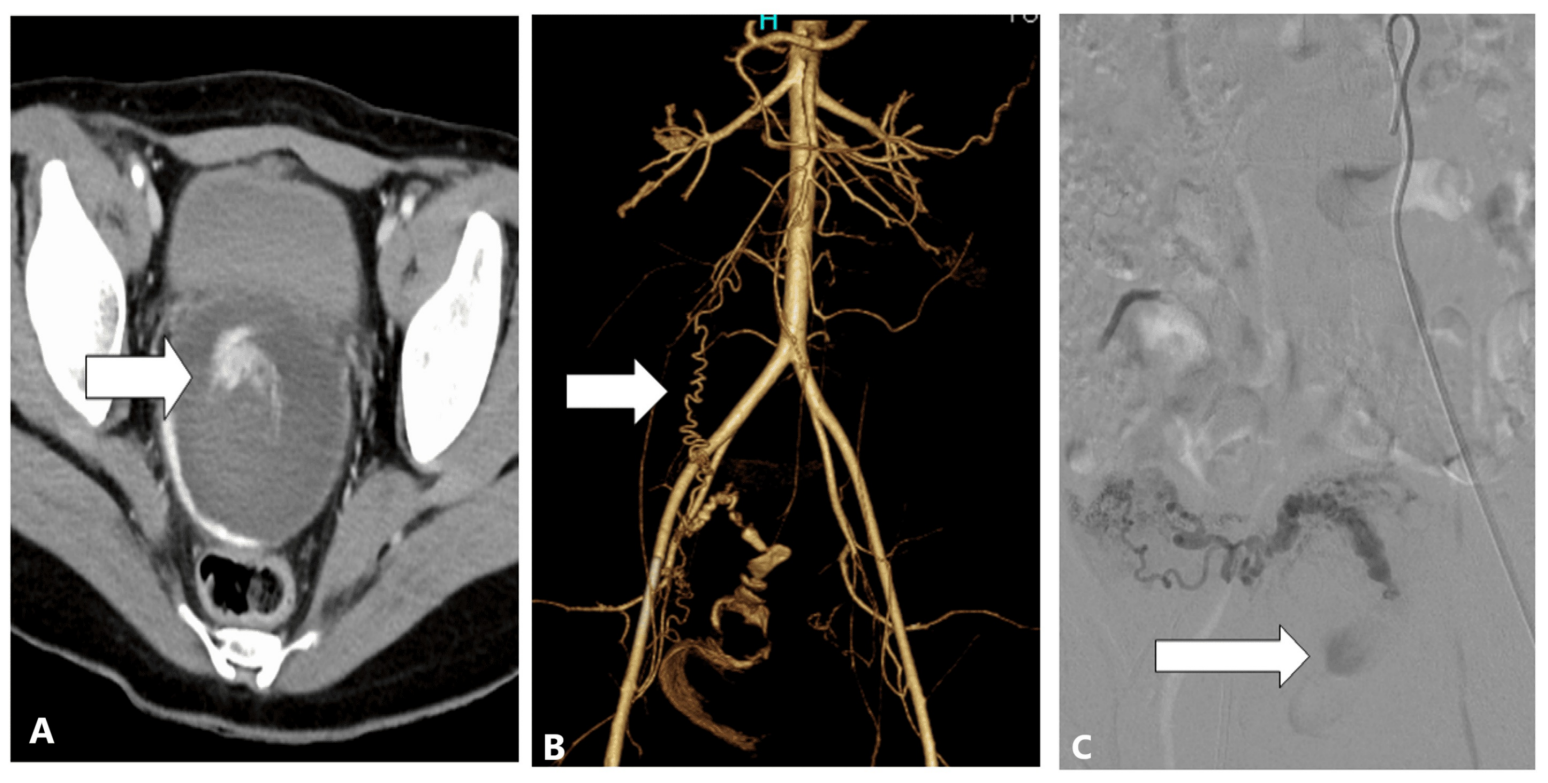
CT, CTA, and ovarian arteriogram of 23-year-old woman with postpartum bleeding within 24 hours of vaginal delivery. (A) A contrast-enhanced CT image shows active contrast extravasation in the uterus (arrow). (B) A computed tomography angiogram reveals a visible right ovarian artery (arrow). (C) A right ovarian arteriogram shows active contrast extravasation from the right ovarian artery (arrow).
